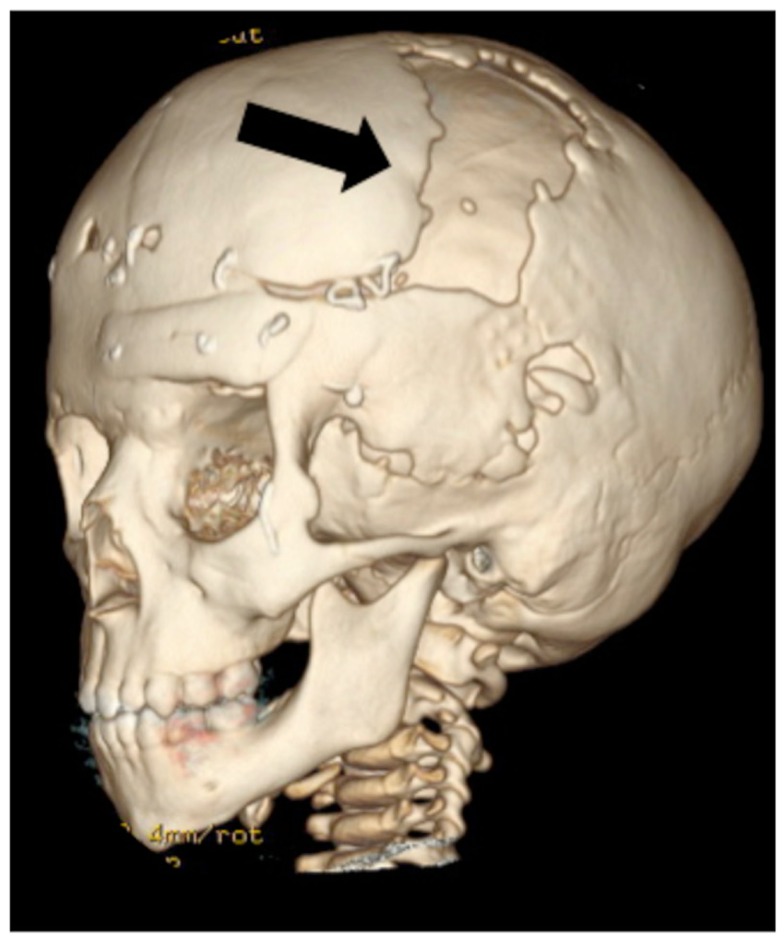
A computed tomography scan demonstrates a large defect (arrow) in the left frontoparietal skull of a 3-year-old boy. This required reconstruction with alloplastic materials or large bone grafts.
